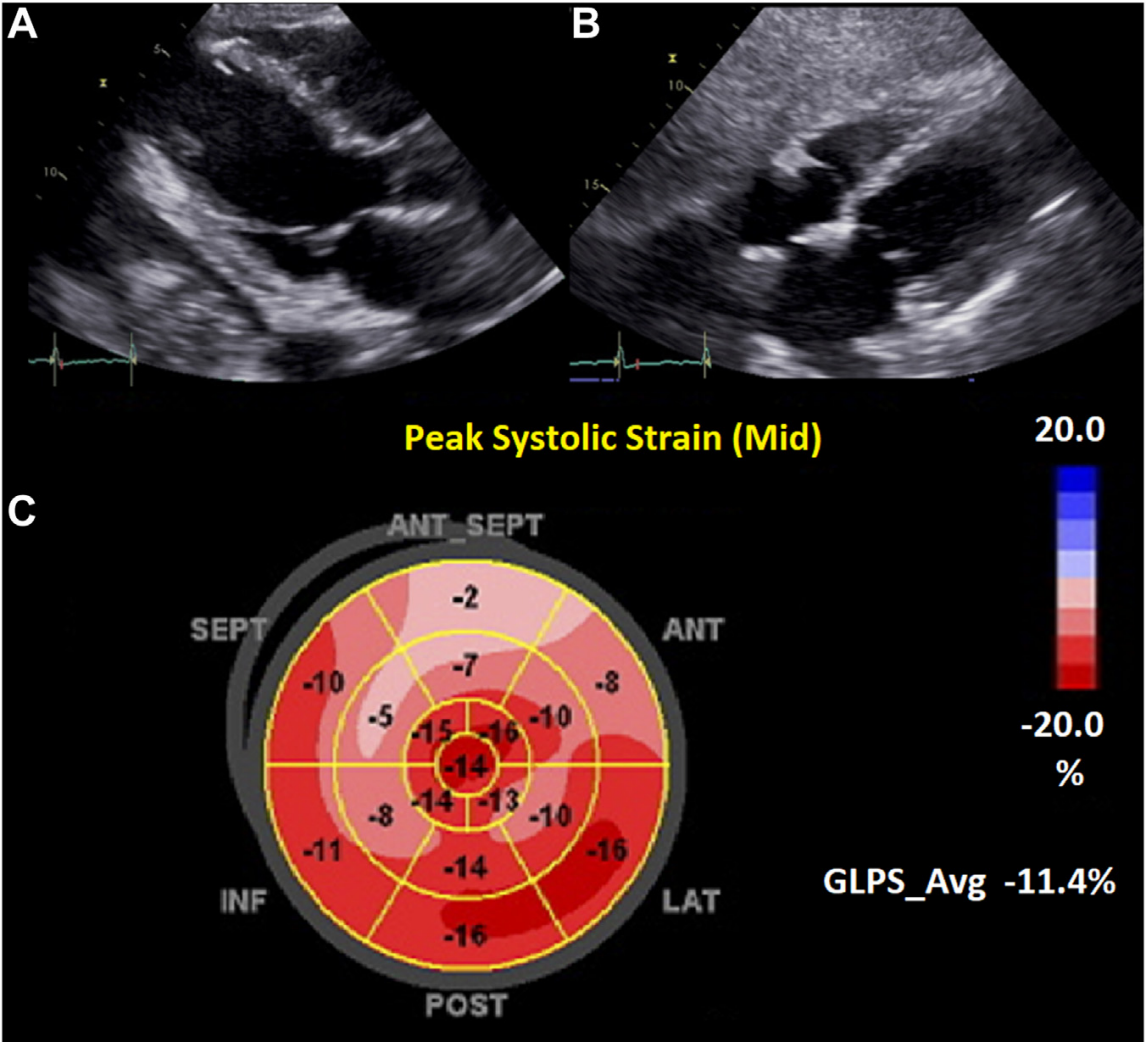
Transthoracic echocardiogram images in patient with chimeric antigen receptor CAR T-cell–induced myocarditis. (A) Parasternal long-axis view; (B) subcostal view showing no significant pericardial effusion; and (C) left ventricular strain demonstrating overall low global strain of –11.4%, and regional abnormalities of the basal to mid anterior and anteroseptum (ANT_SEPT). ANT, anterior; GLPS, Global Longitudinal Peak Strain; HR, heart rate; INF, inferior; LAT, lateral; LAX, POST, posterior; SEPT, septal.
